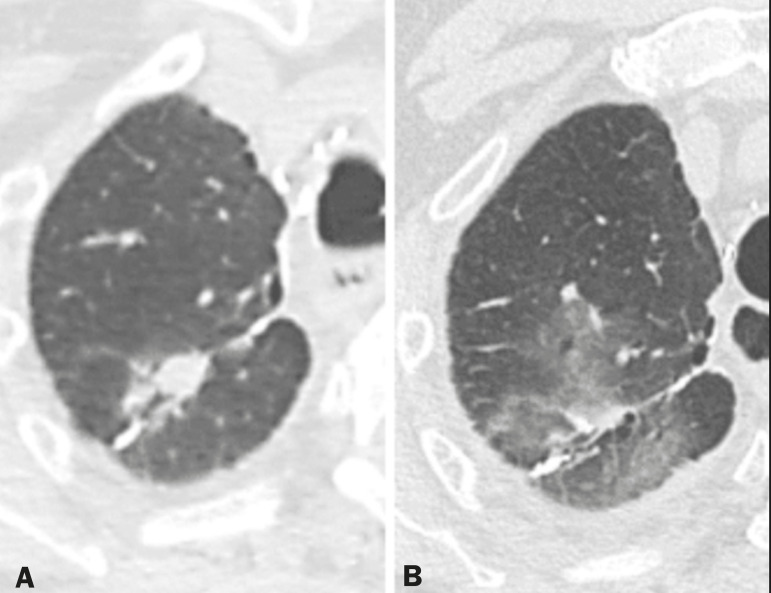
A: CT scan of a patient with a recurrent pulmonary nodule. B: Follow-up CT scan, acquired at 6 months after stereotactic radiotherapy, showing that the patient had developed a linear atelectatic opacity, with a scarring pattern and adjacent ground-glass opacities, at the radiotherapy site.
